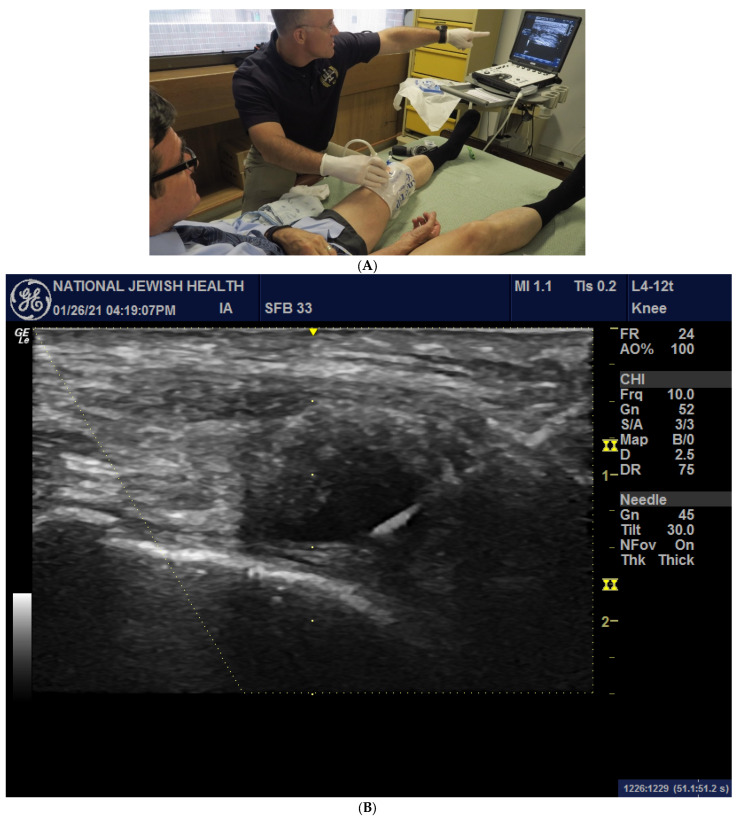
(A) An inflated pneumatic compression device with an image displayed on an ultrasound screen prior to successful knee aspiration of synovial fluid. Image courtesy of Dr. R. Meehan and Dr. R. Scheuring. (B) US image of a study subject during needle insertion, displaying a bright 20-gauge needle entering from the upper right-hand corner of the image with the tip placed within the intra-synovial space (dark anechoic region) during inflation and prior to injecting IA HA product.
